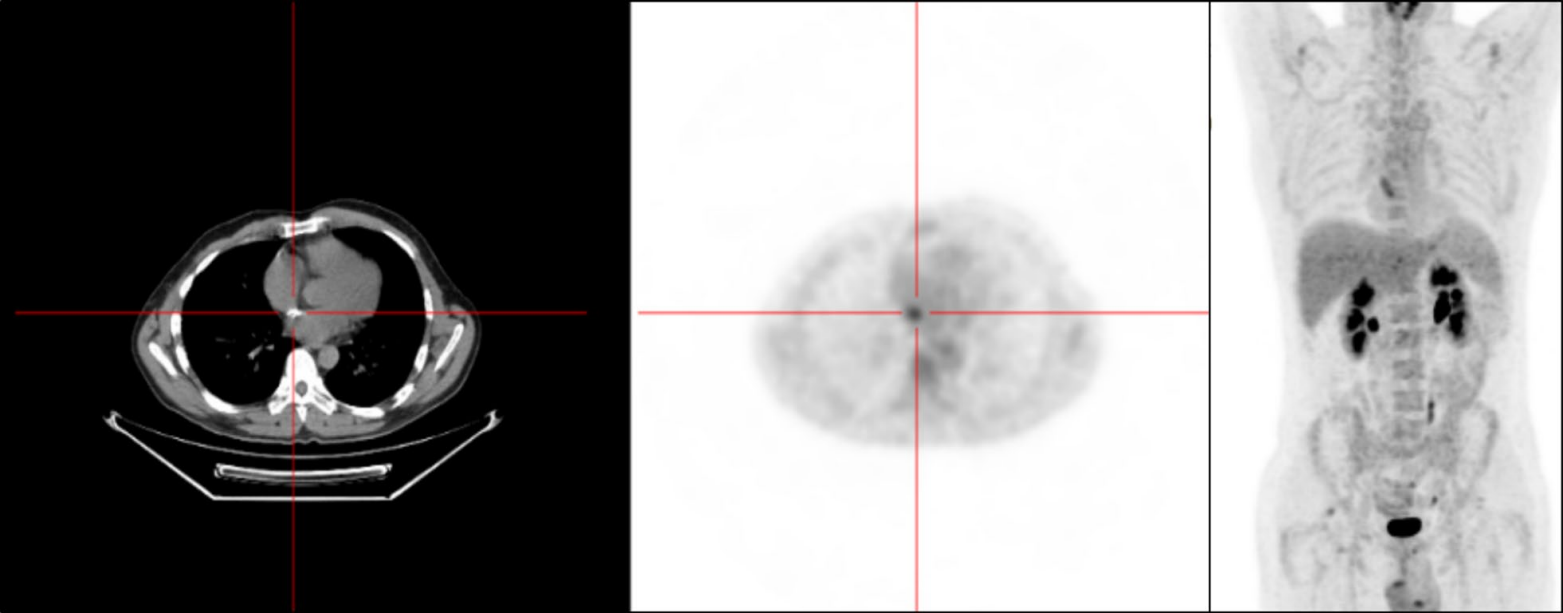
The 18F-fluorodeoxyglucose gated cardiac computed tomography (18F-FDG-PET/CT) imaging showing hypermetabolism hyperactivity around the interauricular septum
